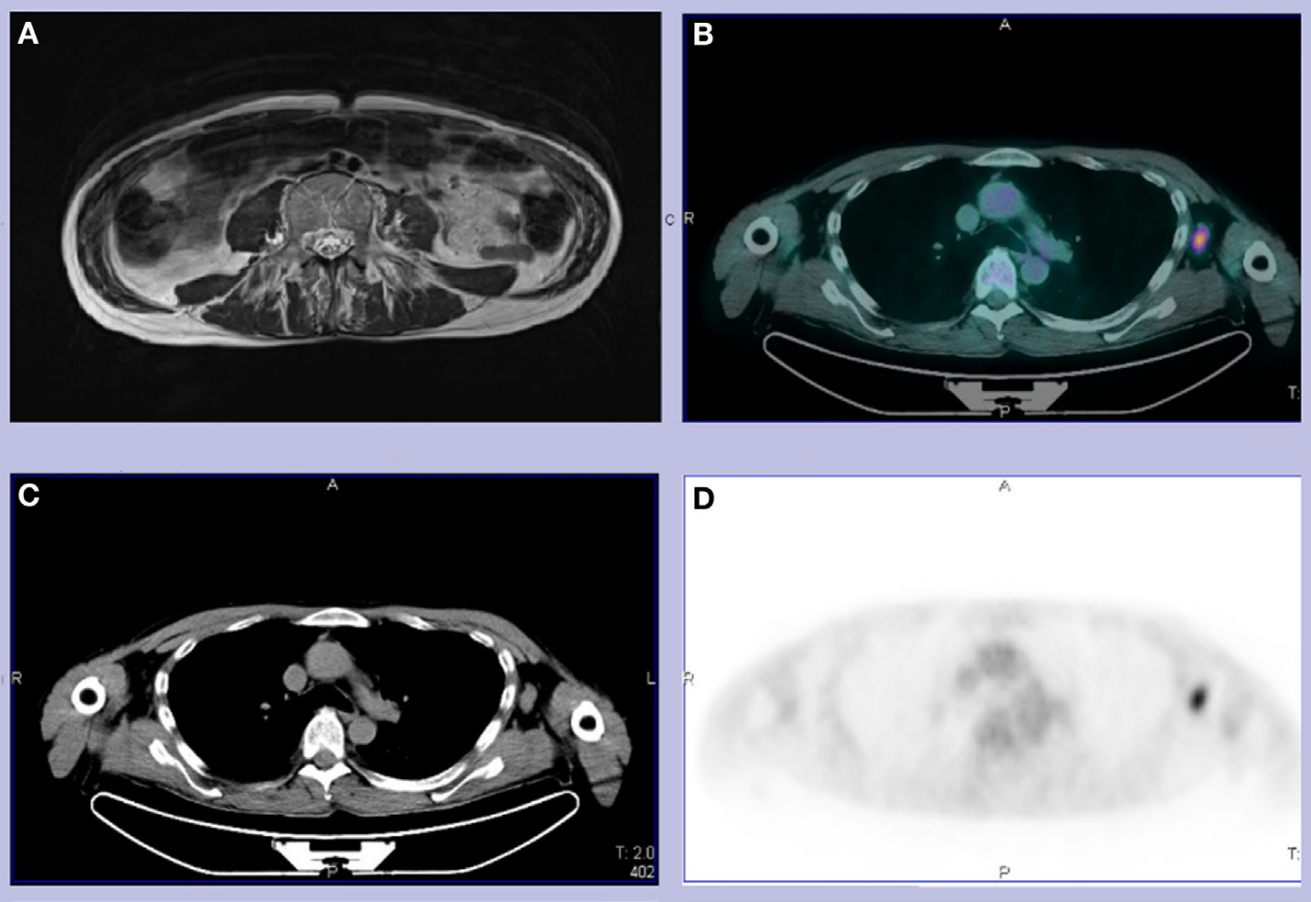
(A) T2 abdominal MRI revealing left psoas and bilateral paravertebral muscles edema and fatty substitution. (B–D) Total body CT-PET showing a hypermetabolic left axillary lymph node. We did not use copyrighted material from other sources (including the web).
